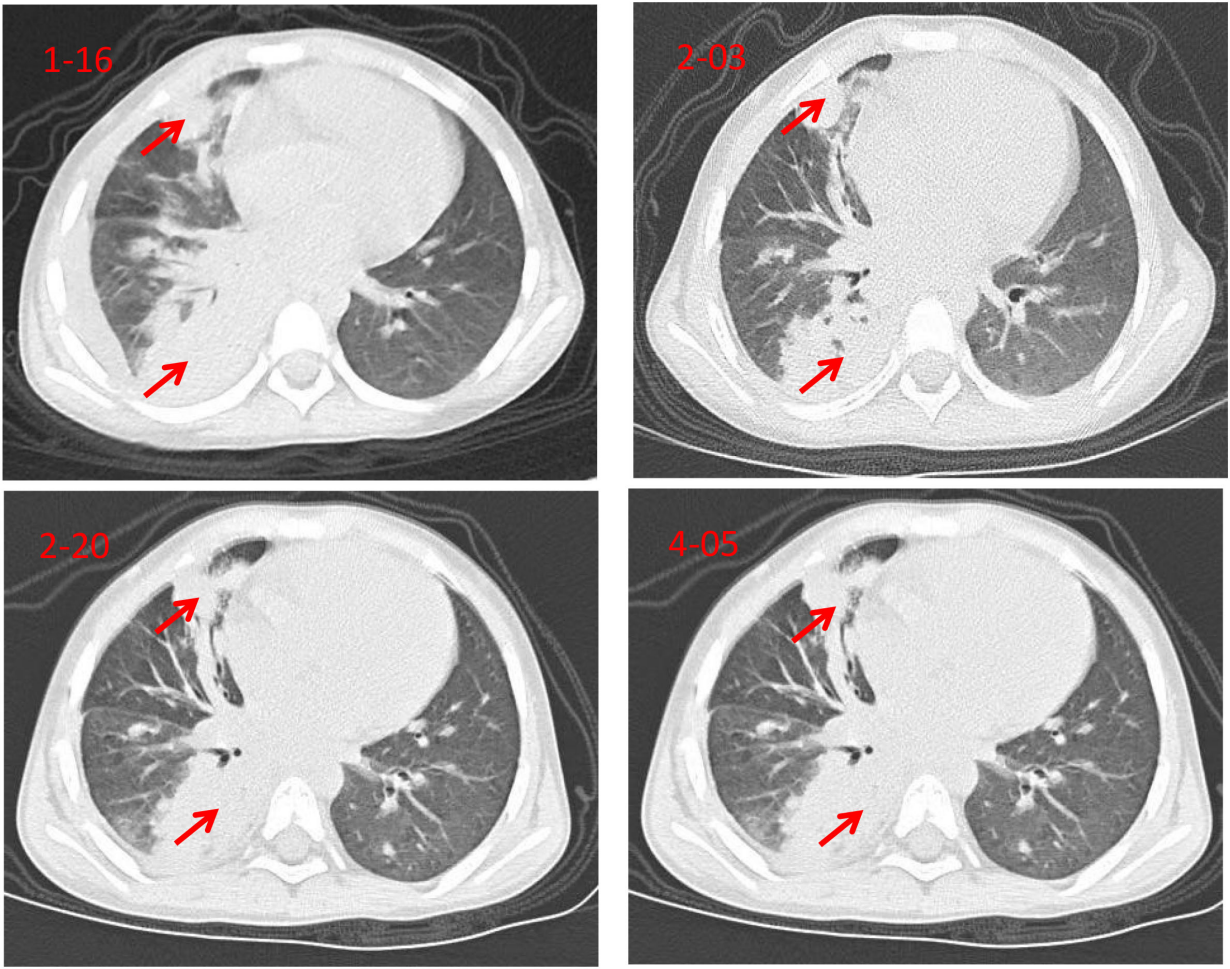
Serial chest CT scans (red arrows indicate bilateral pulmonary consolidation, right pleural effusion, and mediastinal lymphadenopathy; no significant improvement in lesions over time).
